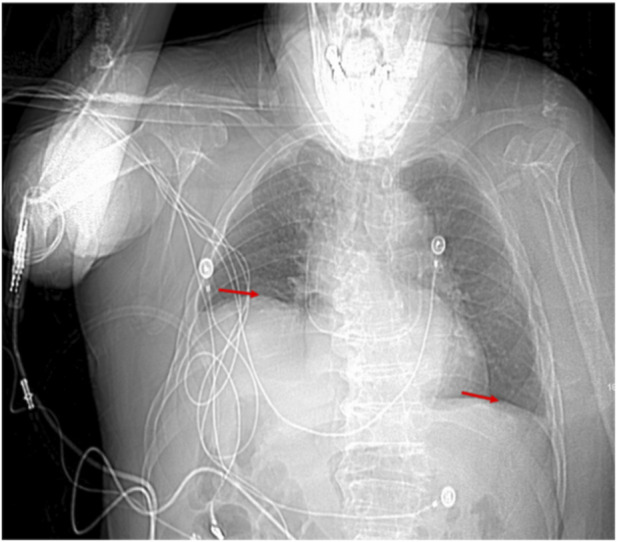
Chest CT (scout view) on postoperative day 5 showing further elevation of the right hemidiaphragm.
